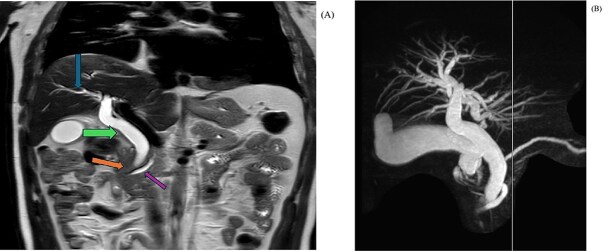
MRI coronal T2 haste (A) showing abrupt cut-off of the distal CBD with a dilated common bile duct, mild degree of intrahepatic biliary dilatation and mild dilatation of the pancreatic duct. MIP image (B) shows the cut off at the distal CBD, with severe dilatation of the extrahepatic and intrahepatic biliary tree, as well as dilated pancreatic duct.
